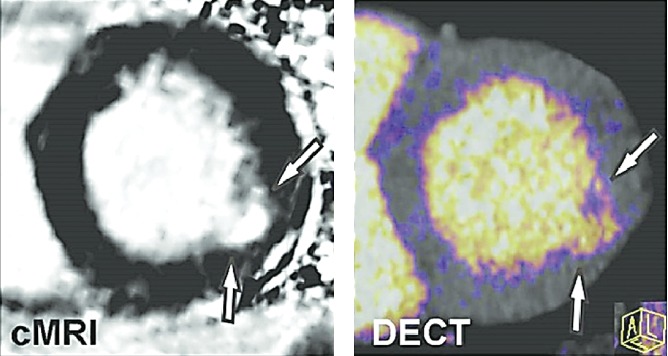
Cardiac magnetic resonance imaging (cMRI) and dual-energy computed tomography (DECT) imaging of myocardial infarction. Iodine color map reconstructed from a DECT image demonstrates good agreement when compared with delayed enhancement cMRI of a subject with prior myocardial infarction of the lateral wall. Similar to cMRI, DECT offers the potential to assess myocardial infarction as well as myocardial salvage through the use of pre- and postcontrast techniques [45].
